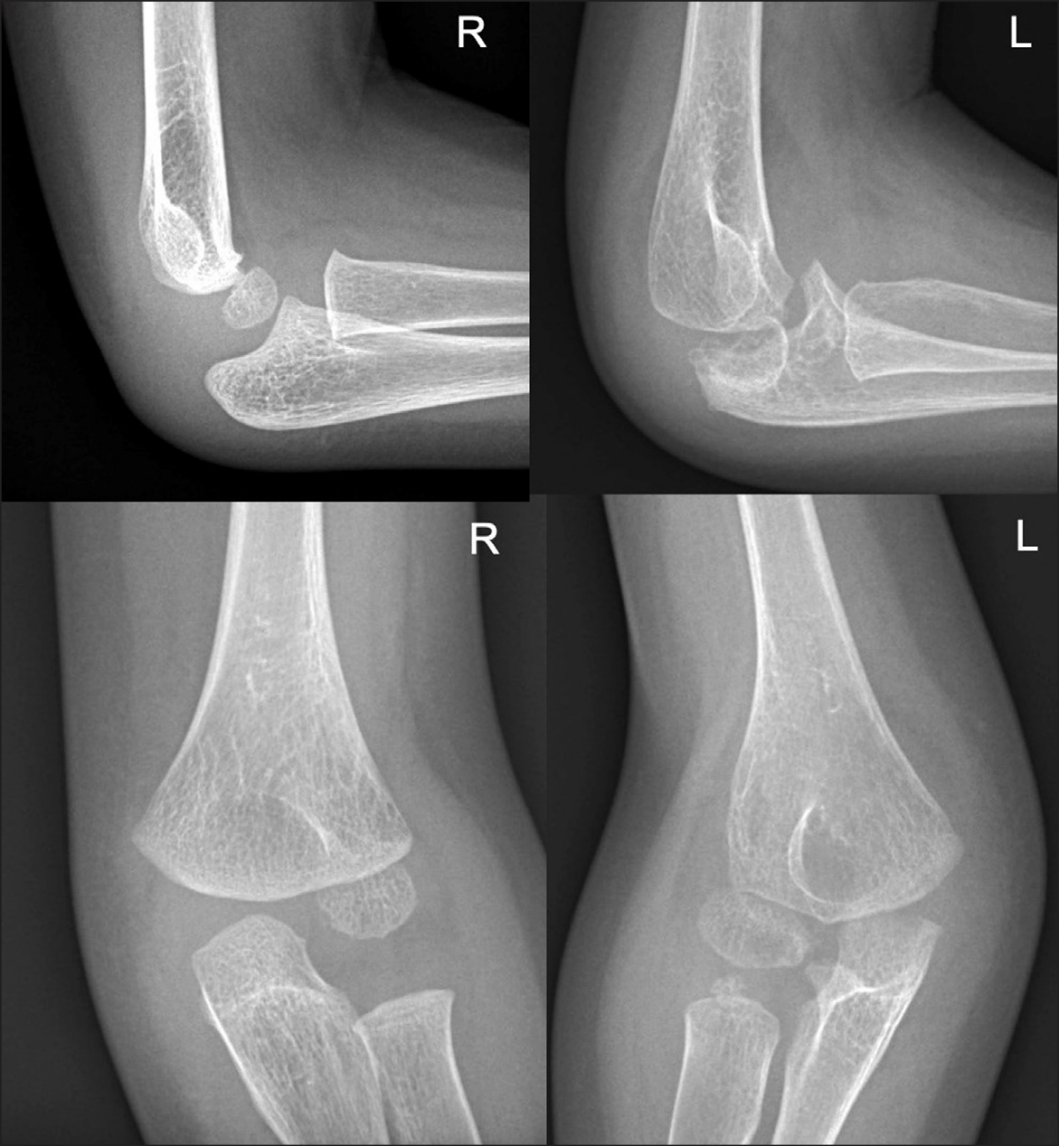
The radiographs of elbows show a significant osteolysis of the left elbow, leading to joint collapse. Soft tissues around the right elbow are swollen. The right elbow appears normal.
